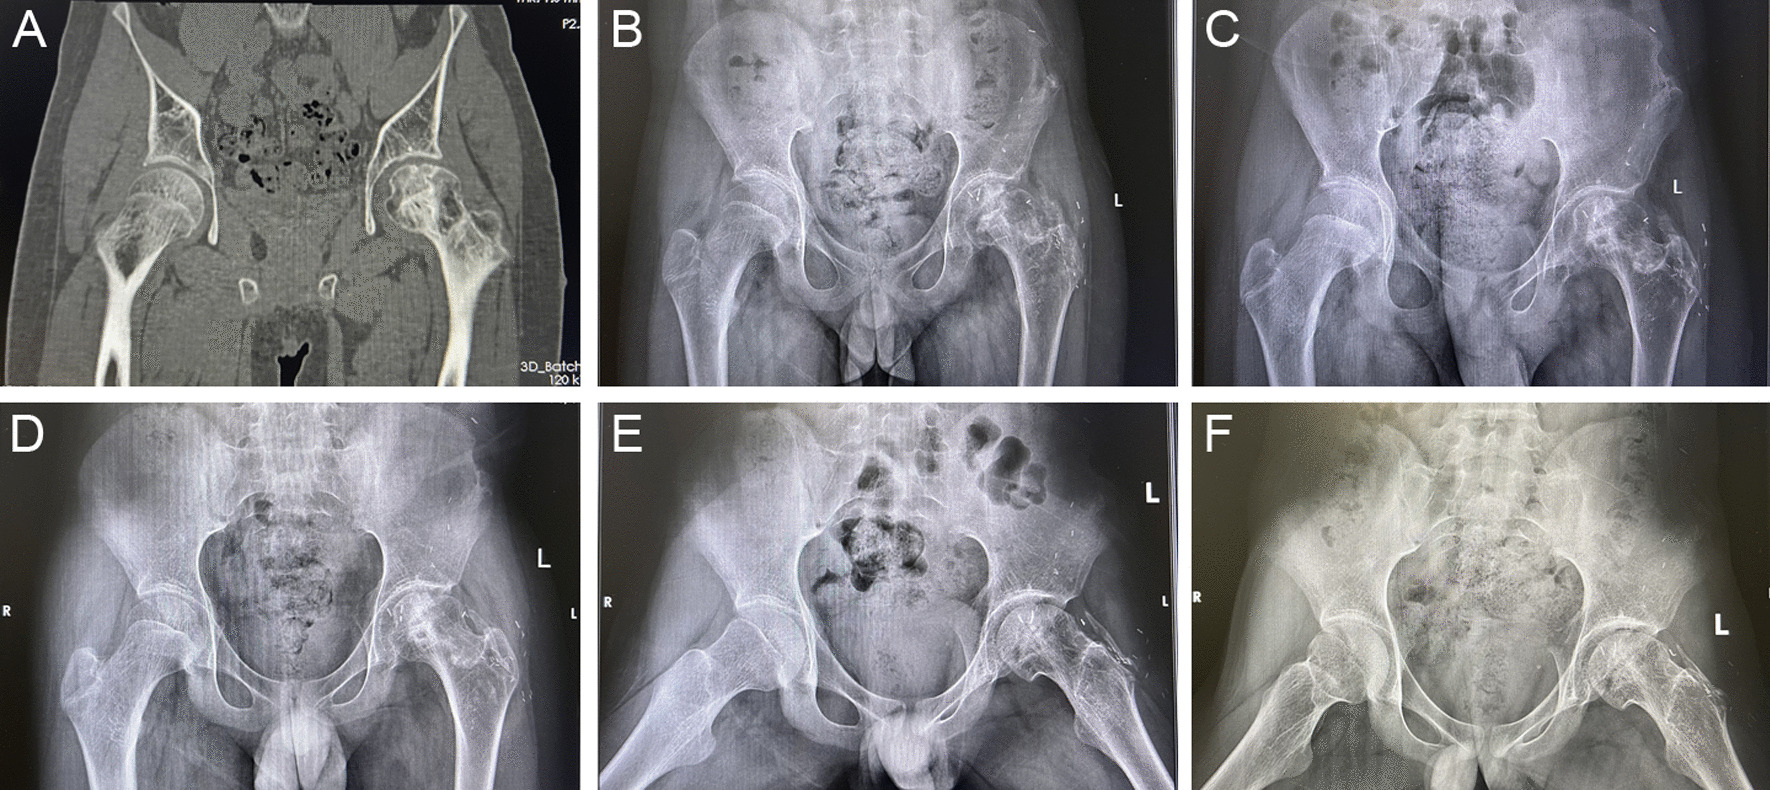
Case 1: A 12-year-old men patient with ARCO III traumatic ONFH at left received the treatment of retrograde anatomy iliac bone flap transfer. A Preoperative radiograph of CT image: the articular plane is uneven, there is obvious necrosis and osteolysis. B–F The anteroposterior radiograph of the pelvis at 1 months, 3 months, 6 months, 12 months, 24 months postoperatively: During the whole follow-up, the shape of the femoral head gradually became round without progressive collapse, and the joint space was well preserved
